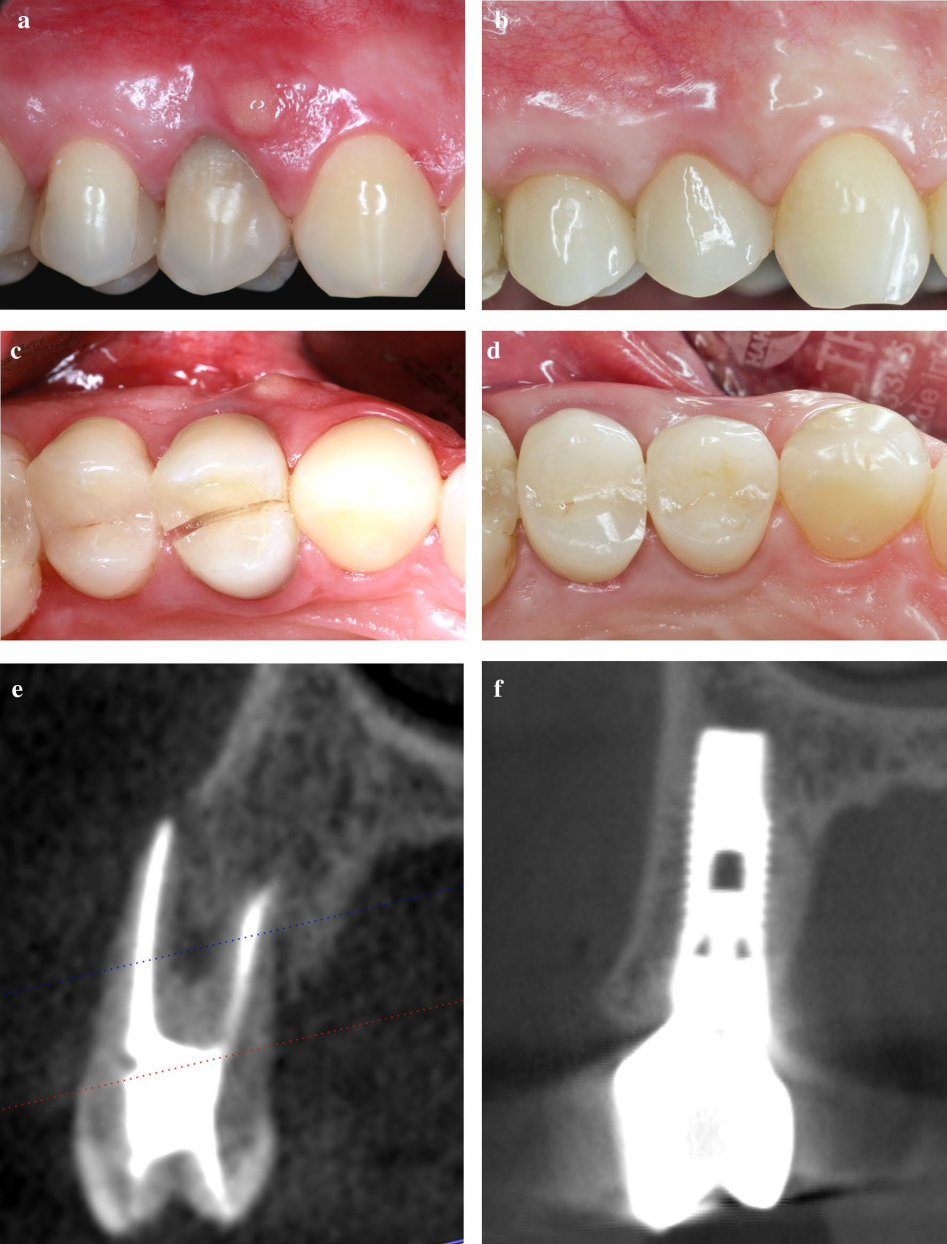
Immediate implant placement in presence of total buccal bone loss and flapless grafting with autogenous bone chips. a Initial clinical situation showing a tooth discoloration after root canal treatment, a slight gingival recession and buccal fistula followed to a crown and root fracture. b 12 years after IIP and buccal defect grafting with autogenous bone chips a reduction of the mucosal recession was observed. c Buccal fistula followed by a crown and root fracture (occlusal view). d 12 years after IIP and autogenous bone grafting a slight resorption of the buccal alveolar contour was observed (occlusal view). e Preoperative CB-CT showing a chronic interradicular lesion combined with a total loss of the buccal bone wall followed to a crown and root fracture. f CB-CT at 12y-follow-up examination shows a complete reconstruction of the buccal bone wall to the level coronal to the implant shoulder
